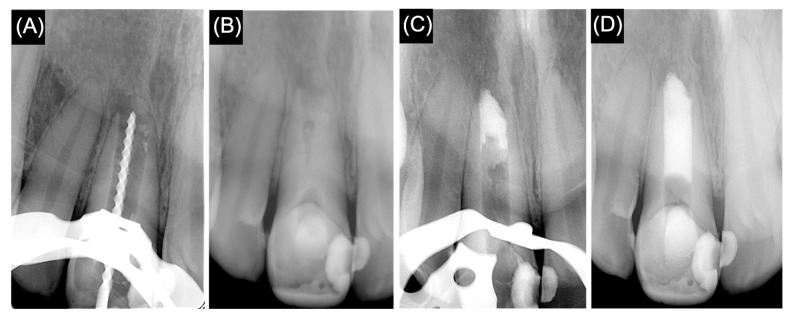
(A) Working length periapical radiograph. (B) Periapical radiograph after the placement of intracanal medicament. (C) Periapical radiograph showing the apical plug. (D) Final periapical radiograph.
